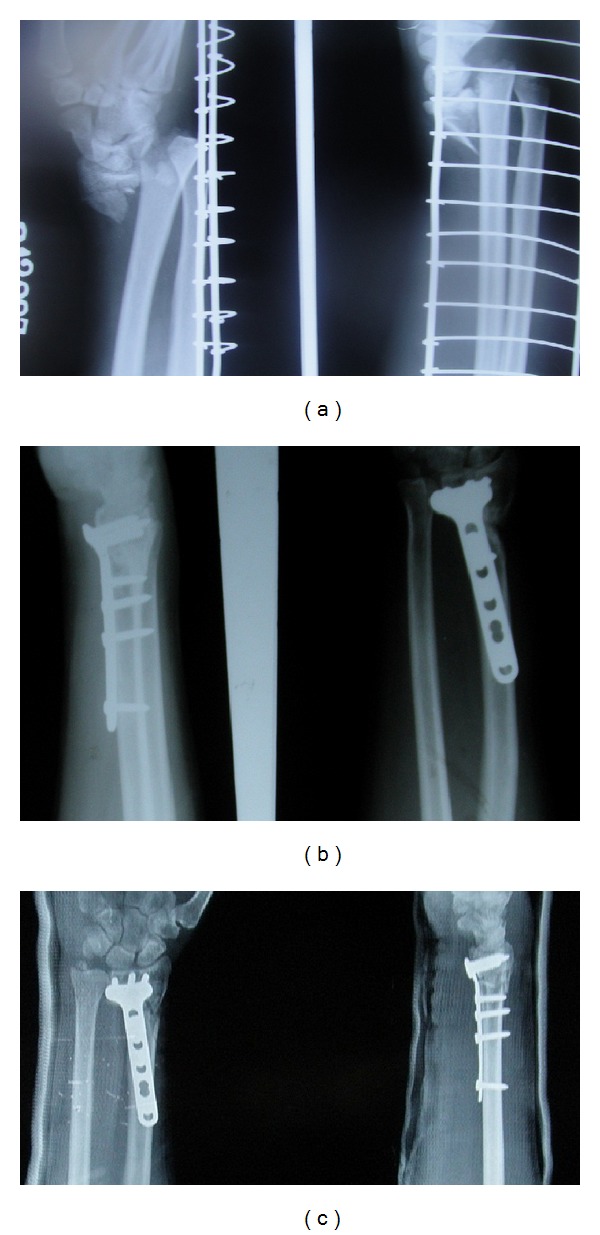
(a) Preoperative radiographs of an AO type A3 fracture of the distal end of the radius in a 53-year-old male. (b) Postoperative radiographs showing adequate reduction. (c) Radiographs at the final followup, showing that the fracture has united and the radiological parameters are maintained.
